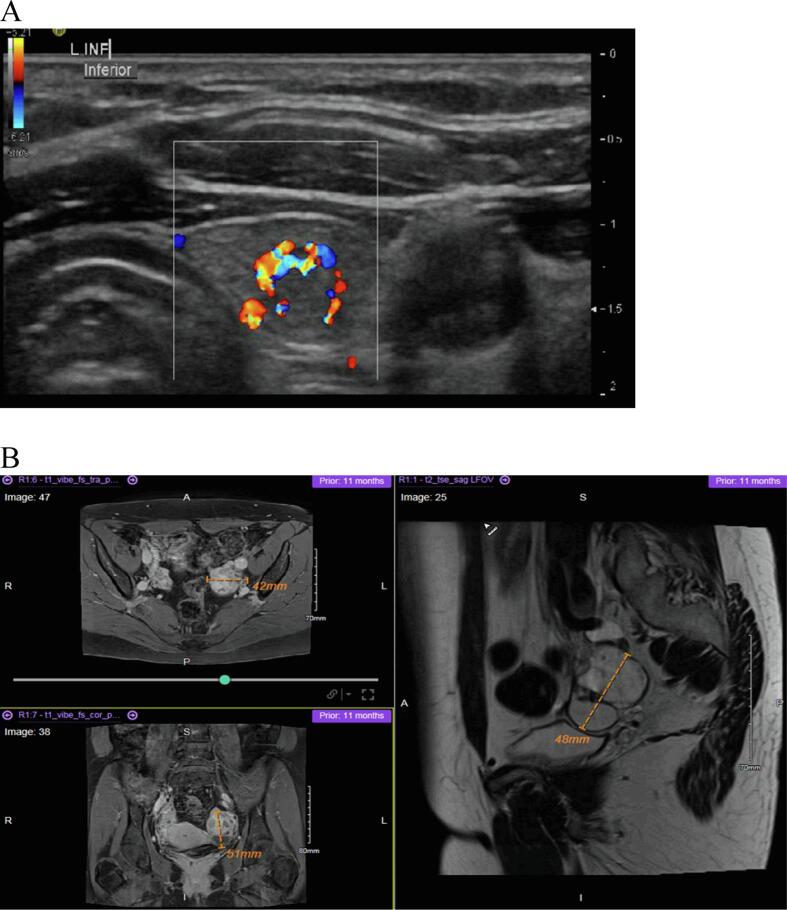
A: Ultrasound image showing a strong doppler blood flow in the ovary (single column width). B: Pelvic MRI with contrast showing a 4.5cm x 2.5cm x 5.0cm T2 hyperintense multiseptated left adnexal mass (full two-column width).
